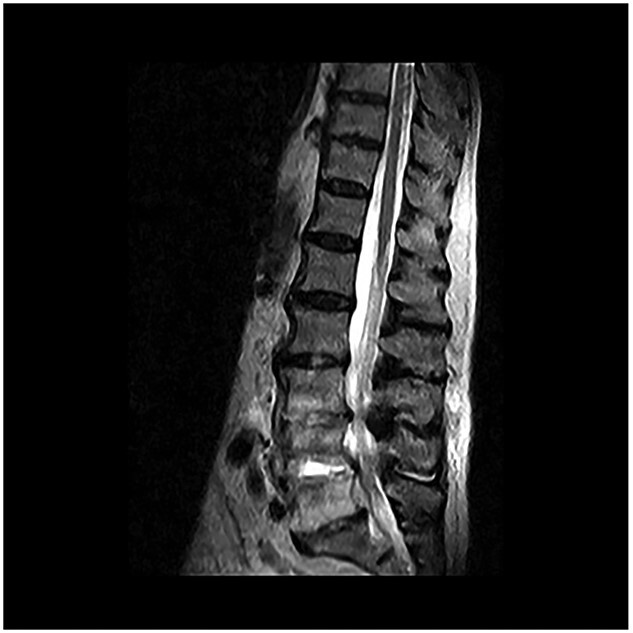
Sagittal T2-weighted magnetic resonance imaging obtained 2 weeks after extracorporeal shock wave lithotripsy for kidney stones complicated by *Escherichia coli* septicemia. Image shows increased signal intensity of L3–L4 and L4–L5 discs and adjacent vertebral bodies. The features indicate ongoing spondylodiscitis.
